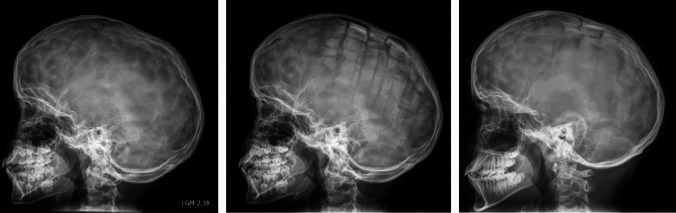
Stable fixation of newly arranged bone segments with PDS sutures. Boy with premature sagittal synostosis, primarily no surgery; skull radiographs as controls revealed digital impressions (left side). Cranial remodelling was performed at 10 years, 5 months (fixation with PDS II^®^). Follow-ups at 5 months post-operatively (middle) and 29 months post-operatively (right) showed no signs of dislocation and demonstrated improving digital impressions
